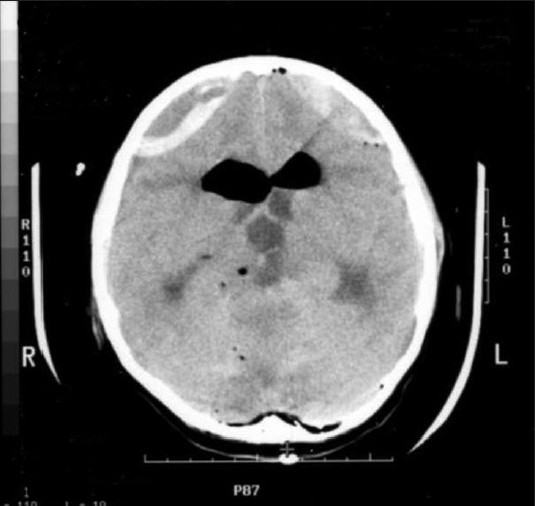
Brain CT showing acute EDHs following reoperation in the bifrontal areas and pneumoventricle in bilateral frontal horns
